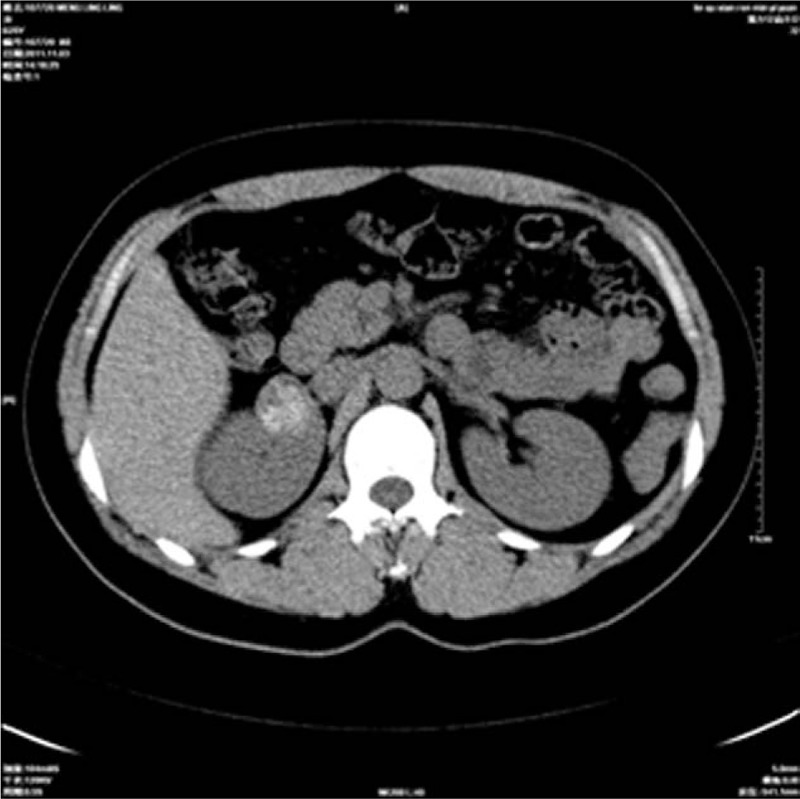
Non-contrast CT demonstrated a heterogeneous mass with obvious calcification and well-defined border measuring about 30 mm in the right kidney. CT = computer tomography.
